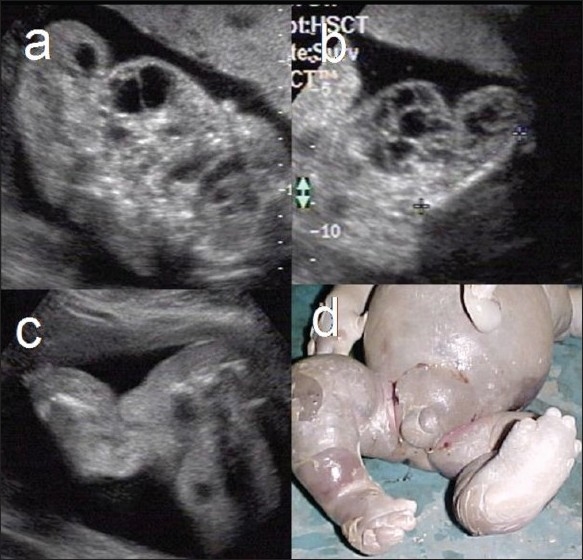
Klippel-Trenaunay-Weber syndrome. Subcutaneous, septate, cystic space-occupying lesions are seen in the right thigh (a) and left arm (b) regions, with extensive subcutaneous edema (c) in the left leg. Postabortal picture (d) of the trunk and lower limbs shows extensive edemaPseudo-TORCH syndrome: (AR) Lead clue is cerebral atrophy with periventricular and basal ganglia calcification. Other features include microcephaly, hepatomegaly, splenomegaly, and thrombocytopenia [Figure 9]. Cordocentesis to prove absence of fetal infection and presence of thrombocytopenia is indicated. Fetal MRI would be of help to confirm cerebral atrophy. Differential diagnoses of Aicardi-Goutiere's syndrome and TORCH infection may be considered.
